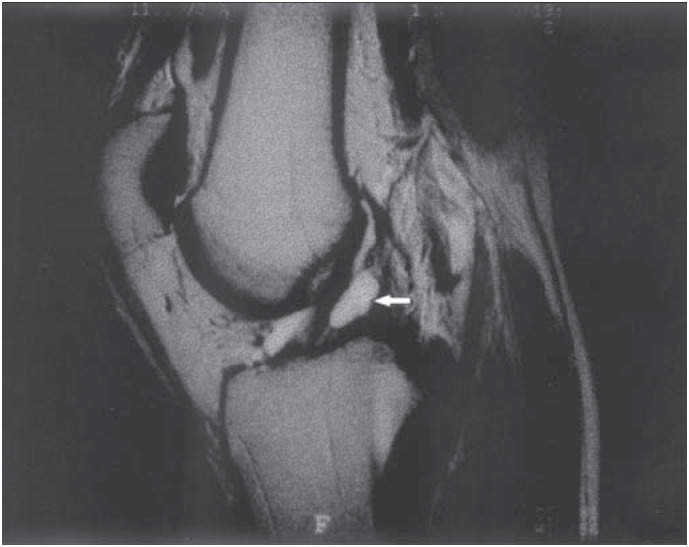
Magnetic resonance imaging of the patient's left knee. White arrow points to the anterior cruciate ligament ganglion.
